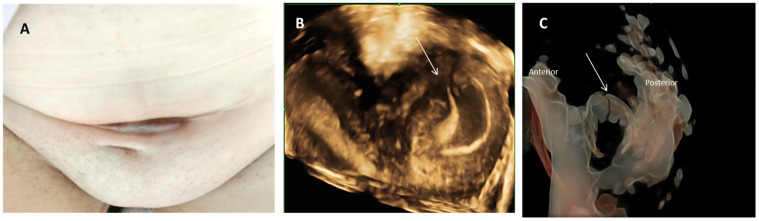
Clinical appearance and ultrasound evaluation: (A) Clinical exam at admission revealed in the middle portion of the Pfannenstiel scar a complex mass with a violaceous color. (B) Three-dimensional transvaginal ultrasound revealed a unicornuate uterus and a thick-walled left pelvic mass suggestive of hematometra in a non-communicating left horn (white arrow). (C) Silhouette ultrasound reconstruction of the uterus describing a fistulous communication between the left uterine horn and the anterior abdominal wall mass.
